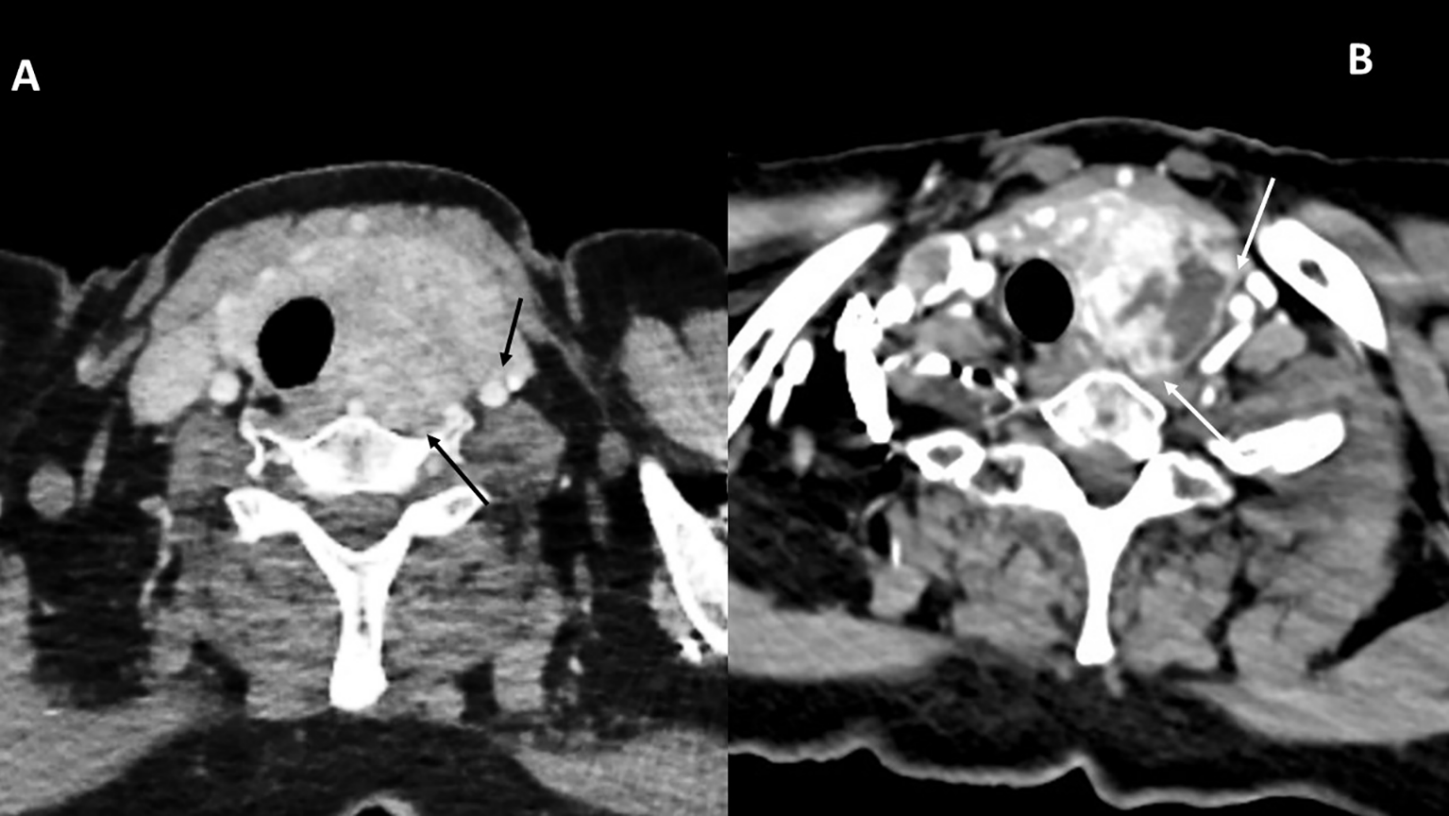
CT scan images for patient 1, before systemic therapy (A) and 3 months after discontinuation of both drugs (B). The involvement of the prevertebral fascia and common carotid artery classified this tumor as initially unresectable (black arrows, A). Tumor response is indicated in B (white arrows).
